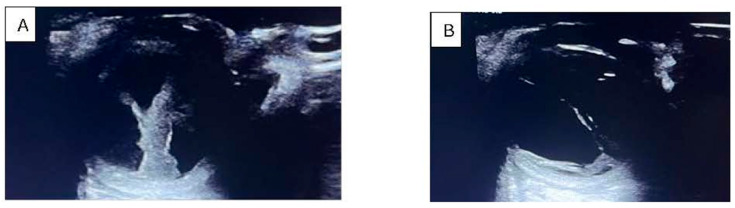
B-scan ultrasound showing (A) inflammatory membranes, dense hyperechogenic lesions, and inflamed periocular tissue; (B) tractional inflammatory membranes. The posterior segment cannot be visualized because it is obscured by the vitritis. Photo: Malciolu-Nica M.A. Patient has consented to the publication of these photographs.
